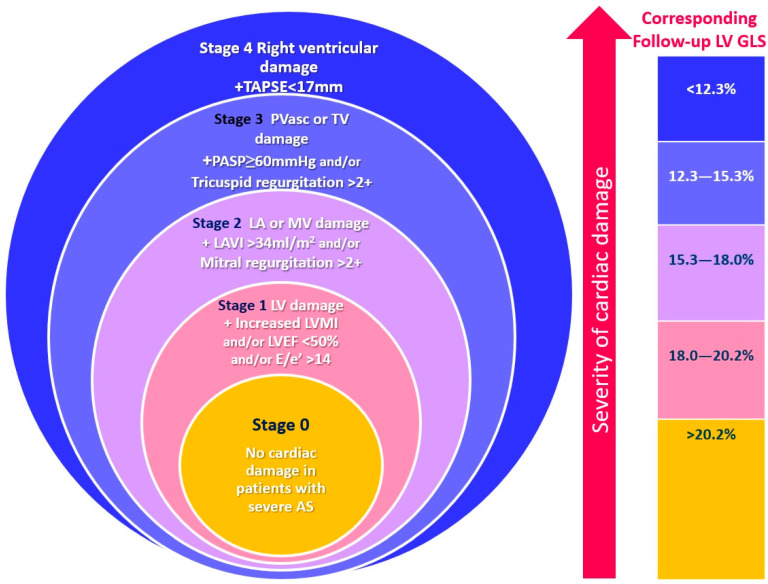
Stages of cardiac damage in severe aortic stenosis. The figure represents the echocardiography-based cardiac damage staging system that was applied before and after TAVI. AS, aortic stenosis; GLS, global longitudinal strain; LA, left atrial; LAVI, left atrial volume index; LV, left ventricular; LVEF, left ventricular ejection fraction, LVMI, left ventricular mass index; MV, mitral valve; PASP, pulmonary artery systolic pressure; PVasc, pulmonary vasculature; TAPSE, tricuspid annular plane systolic excursion; TR, tricuspid regurgitation; TV, tricuspid valve.
